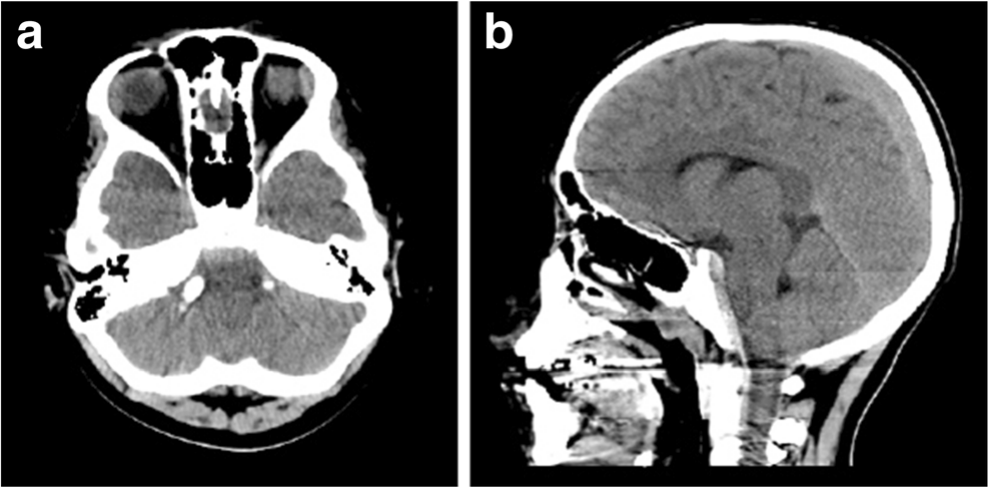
a Axial CT head demonstrates retroclival hematoma. b Mid-sagittal CT head demonstrates retroclival hematoma
